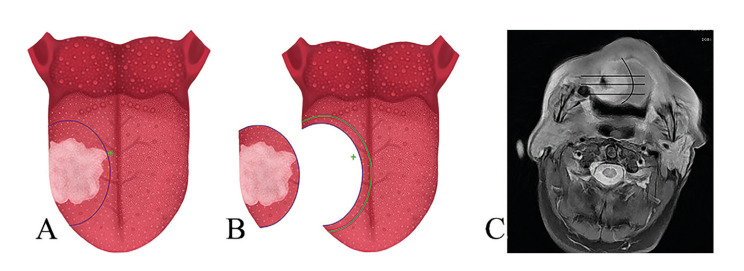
A) Resection of a tongue tumor, with the asterisk (*) indicating the area at risk of proximity to tumor margins. B) Re-resection of tumor bed with extension into the positive area, including extensive healthy tissue. C) MRI and CT scan revealing irregular tumors.
